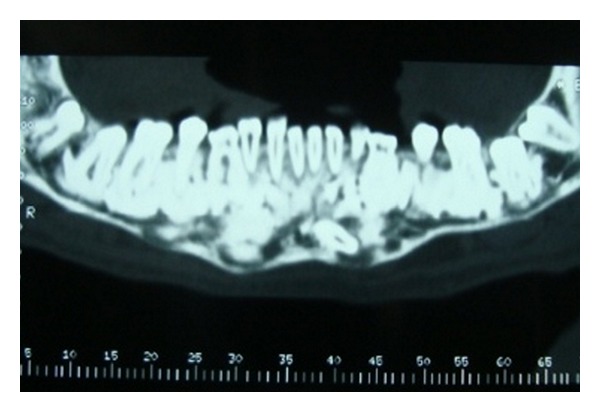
CT scan showing panoramic like reconstruction (Case 1).
